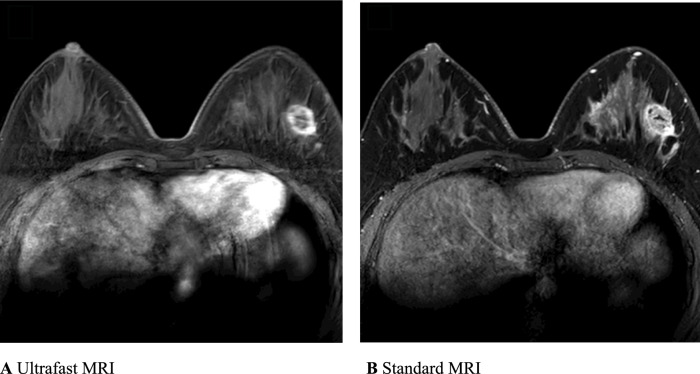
Ultrafast and standard MRI in a 30-year-old woman with triple-negative invasive ductal carcinoma. A Ultrafast MRI taken 16.8 s after contrast agent injection. B Standard MRI taken 58.8 s after contrast agent injection. Both images show an oval-shaped, irregular marginated, rim enhancing mass in the left outer breast. For three-dimensional tumor segmentation, the entire enhanced tumor margins were drawn from top to bottom of each tumor in axial views of U2 phase postcontrast ultrafast MRI and the initial phase postcontrast standard MRI. Among the 14 ultrafast images, U1 is the first phase in which the signal intensity of the hotspot region of interest of the tumor is 10% higher than the average signal intensity of unenhanced images. U2 is the immediate next phase of U1 and the well-established tumor enhancement time
